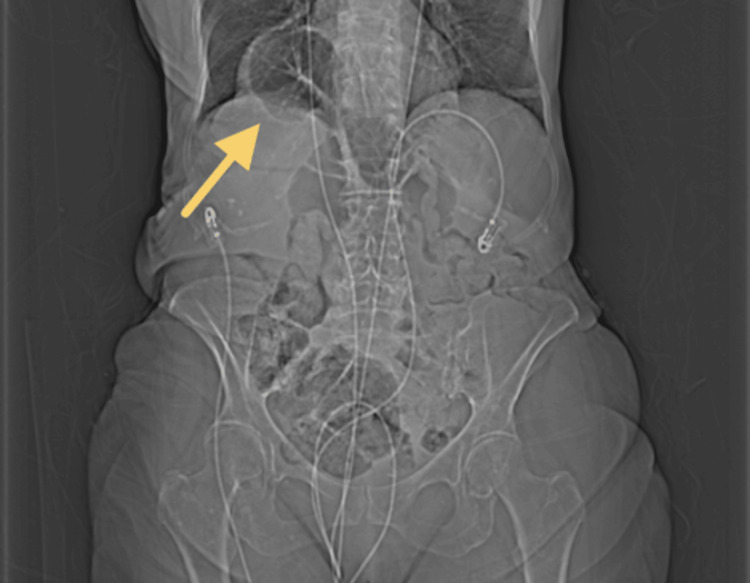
Abdominal X-ray demonstrating Morgagni hernia findings The arrow indicates a short loop of transverse colon herniating through a diaphragmatic defect into the thoracic cavity
